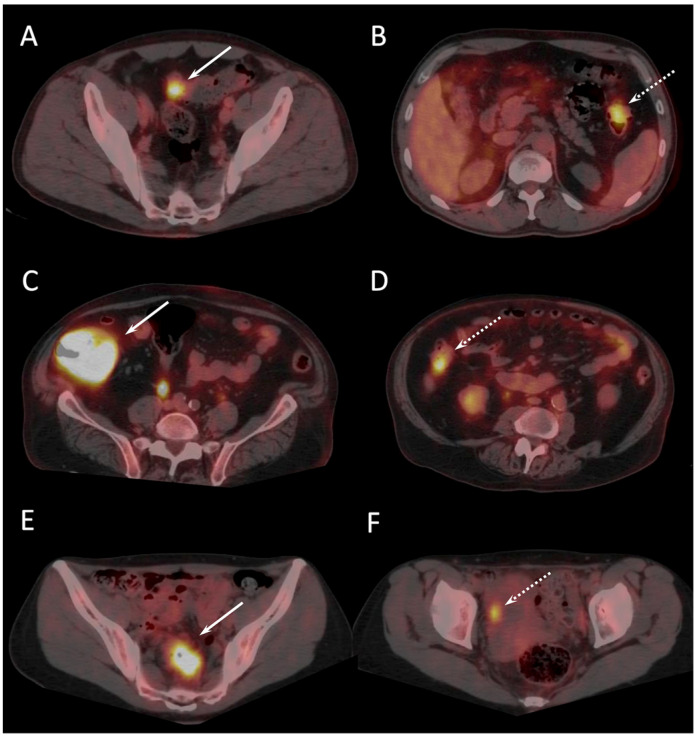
Presurgical 18F-FDG PET/CT results of 3 patients with presumed localized CC (arrows) showing additional colon lesion or visceral metastasis not visualized at conventional staging (dotted arrows). 57-old year man with (pT3) sigmoid primary (A) and synchronous invasive carcinoma (T2) of left colon (B). 74-old year man with 11 cm (pT3) tumor of the caecum (C), and adenoma with high grade dysplasia of the right colon (D). 46-old year woman with incomplete colonoscopy due to a sigmoid tumoral stenosis (pT3) (E), and right ovary metastasis (F).
